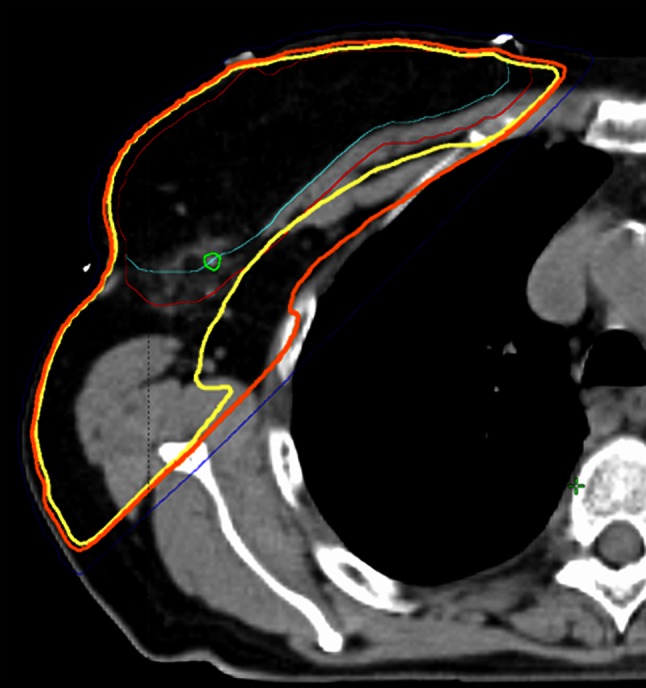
Radiotherapy planning CT of a study patient after lumpectomy and SLNB with an elective radiation dose at the surgical clip. The breast tumor of this patient was located in the mediocaudal quadrant of the breast; the tumor bed is not visible on this CT image. The *yellow line* contouring the breast represents the 95 % isodose, i.e. all spots on this line received at least 95 % of the prescribed dose; the *orange line* represents the 90 % isodose; the *dark blue line* represents the 50 % isodose; the *light blue line* represents the clinical target volume of the breast; and the *red line* represents the planning target volume of the breast. The surgical clip at the SLN site is contoured by a *green line*. *CT* computed tomography, *SLNB *sentinel lymph node biopsy, *SLN* sentinel lymph node
